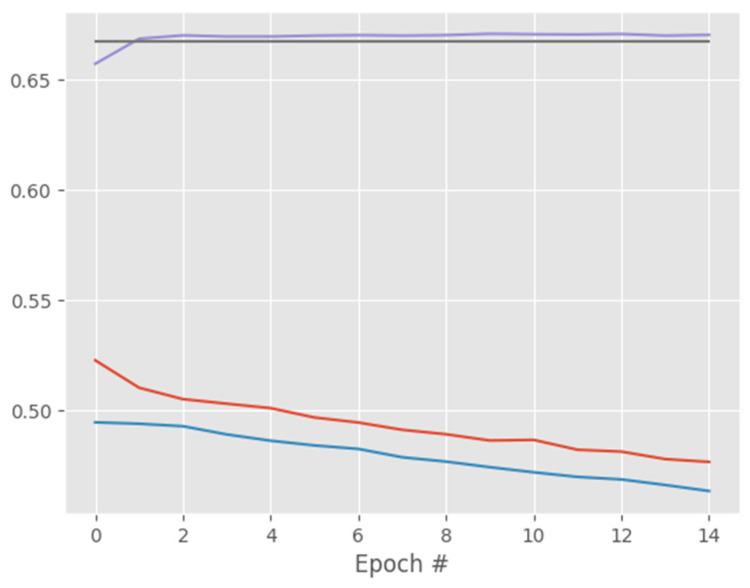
ResNet50 training and validation performance for X-ray images.
